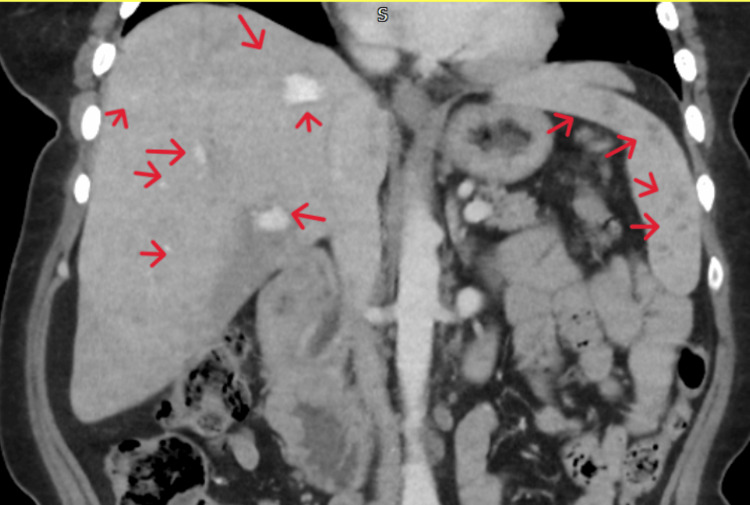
CT abdomen and pelvis showing hepatomegaly with multiple focal lesions in the liver and spleen, no abdominal or pelvic lymphadenopathy
